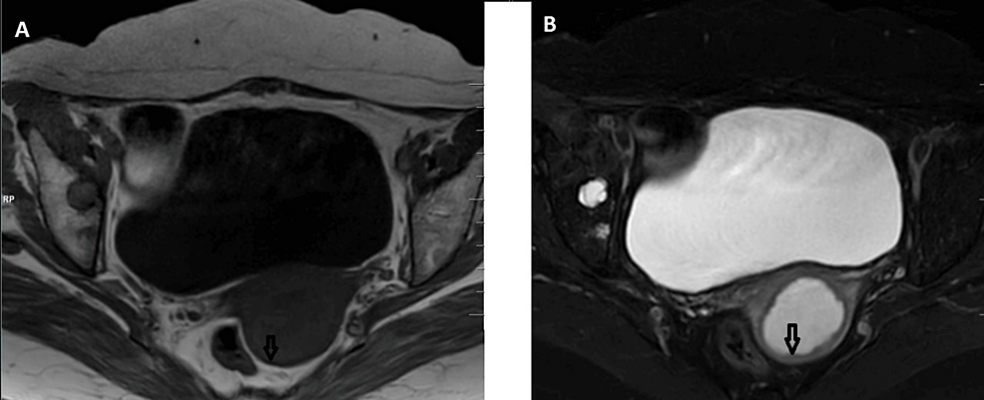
MRI pelvis T1 (A) and T2-FS (B) axial sections of the pelvis showing thinned-out endometrium. MRI: magnetic resonance imaging; FS: fat saturated
